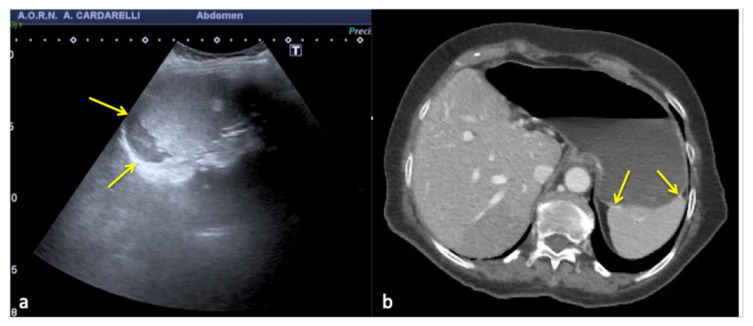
Transverse US image of the left hypochondrium (a) shows a large hypoechoic area misinterpreted as splenic hematoma (arrows) in a 31-year-old woman investigated for trauma. On CT scan (b) it appears to be a gastric fundus distended by fluid (arrows).
